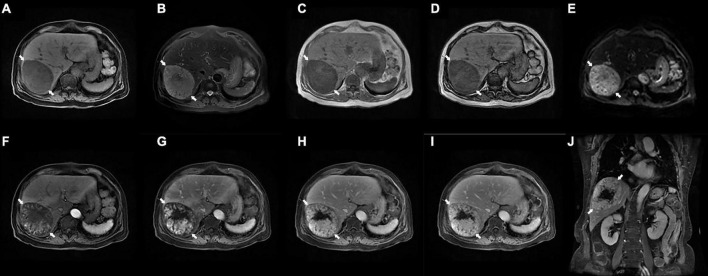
Preoperative enhanced magnetic resonance imaging (MRI) examination. (A) T1WI shows an ovalshaped hypointense lesion with clear border in the right lobe of the liver (arrows, 94×74 mm). (B) Fat-suppressed T2WI shows a slightly hyperintense lesions (arrows). (C,D) The in-phase (C) and out-of-phase (D) of T1WI demonstrates hypointense speckled signals within the mass (arrows). (E) DWI shows a hyperintense lesions (arrows). (F–J) Enhanced MRI scans showed progressive enhancement of the lesion (arrows) on the early (F) and late (G) arterial phase, portal venous phase (H), delayed phase (I), and coronal view (J), while no enhancement was seen in the necrotic region. MRI, magnetic resonance imaging; T1WI, T1 weighted image; T2WI, T2 weighted image; DWI, diffusion weighted imaging.
